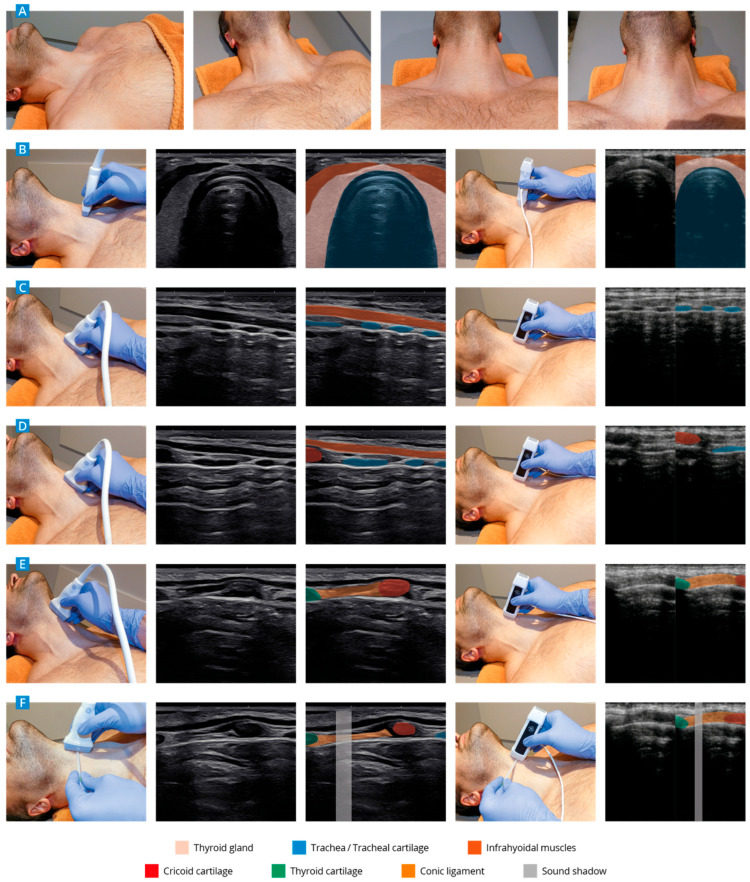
The technique for sonographic identification and indication of the conic ligament with a medium class device is depicted on the left and a pocket device on the right side (based on [14,15]). After positioning (reclination) of the patient (A), the trachea is visuallized in transverse section at the level of the thyroid (B). The probe is turned 90 degrees clockwise for the representation of the tracheal cartilage (C). The probe is moved cranially for visualisation of the cricoid cartilage (D), the thyroid cartilage (D), and the identification of the conic ligament (E). The use of a cannula to mark the conic ligament (acoustic shadow) is depicted (F).
